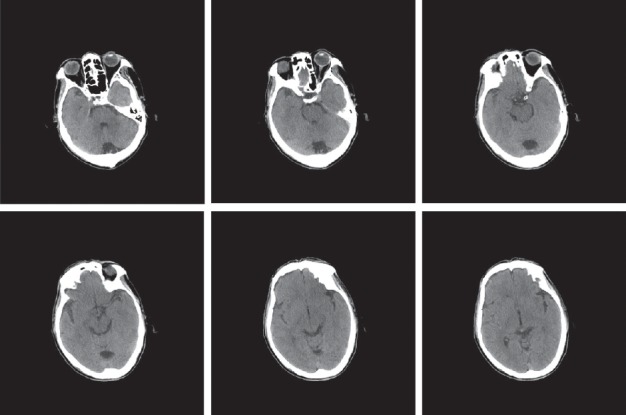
The CT examination in August 2nd. No blood could be seen.
